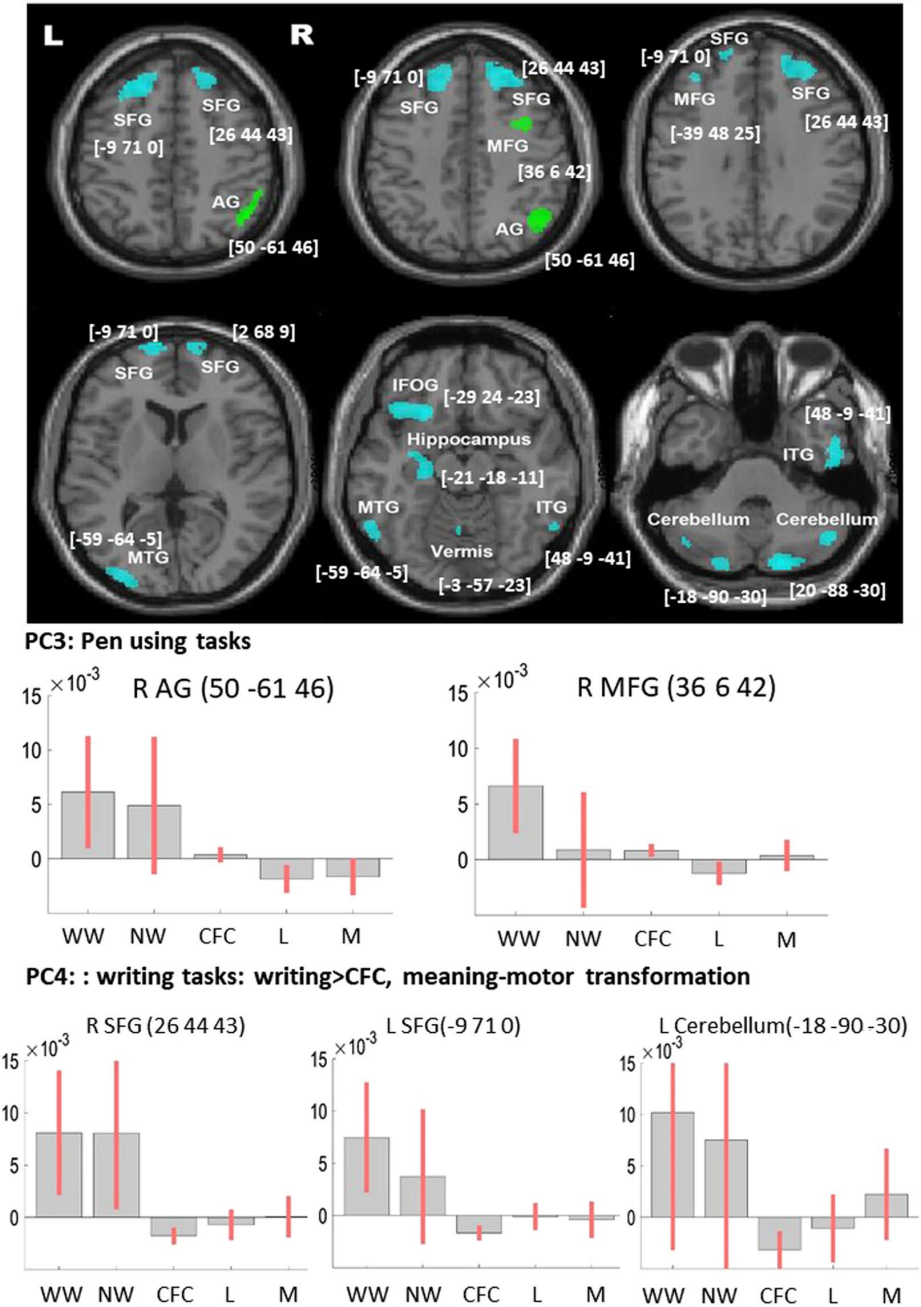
VBM results with PCA component (model 3, N = 267). The VBM results overlaid on the canonical T1 images (SPM), showing lesions associated with the PCA writing specific components. Lesion associated with using pen (PC 3) are presented in green, lesion associated with using pen to produce meaningful symbols (PC 4) are blue. The charts represent effect size (beta) for each writing tasks, the complex figure copy and the average effect size for the language (L) and motor (M) tasks. The error bars are 90% confidence interval of the effect size. Abbreviations: L, left, R: right, SFG, superior frontal gyrus; MFG, middle frontal gyrus; IOFG, inferior orbitalis frontal gyrus; AG, angular gyrus; MTG, middle temporal gyrus; ITG, inferior temporal gyrus, IOG, inferior occipital gyrus; WW, word writing; NW, number writing; CFC, complex figure copy; L, language tasks, M, motor tasks. See Supplementary Figs. 2 and 3 for case examples of lesion on the CT scans.
